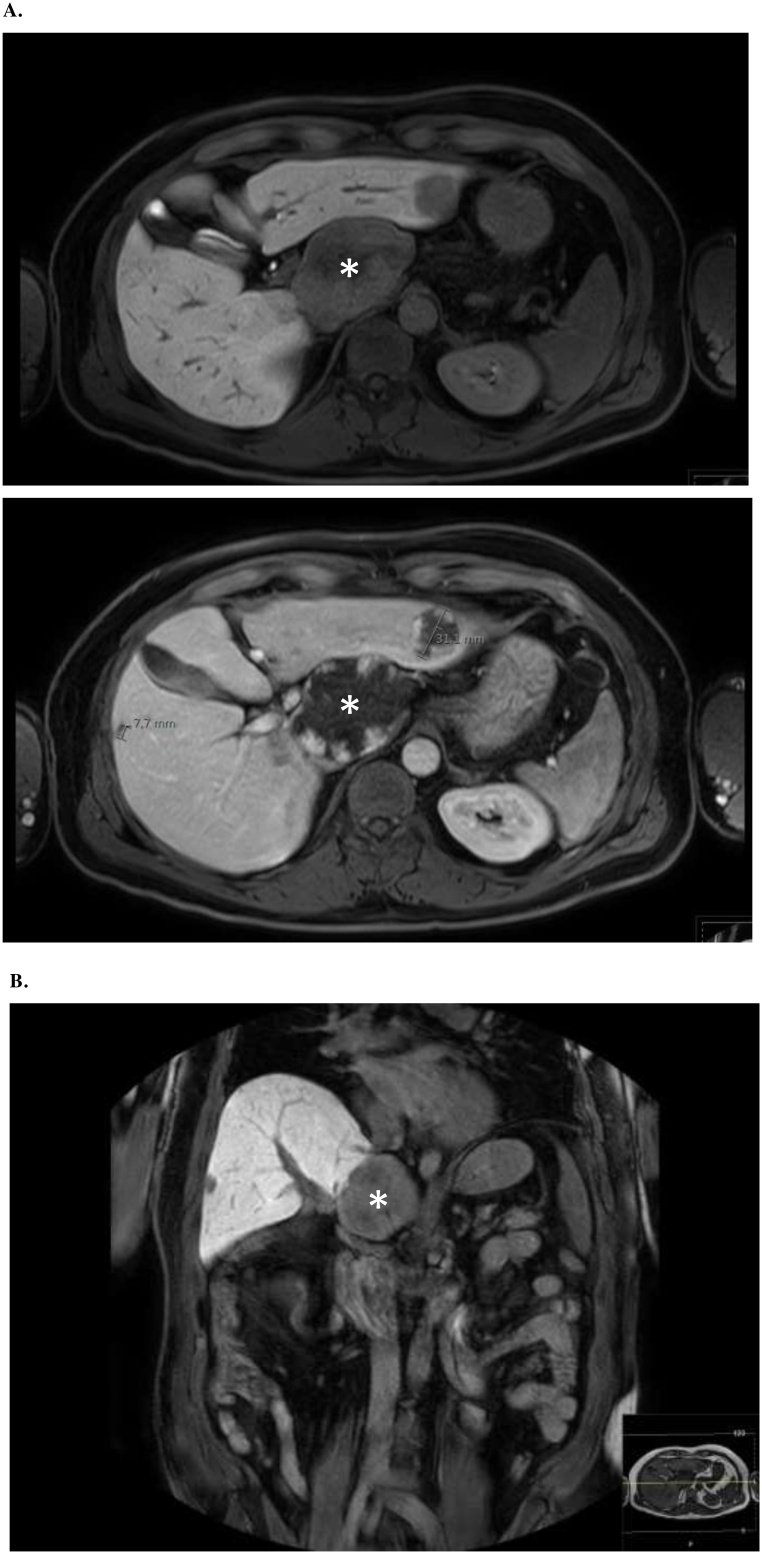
MRI images showing multiple hemangiomas of the liver. The largest HH is located in segment I (*) compressing the inferior vena cava. A: Axial section MRI. B: Coronal section MRI.
